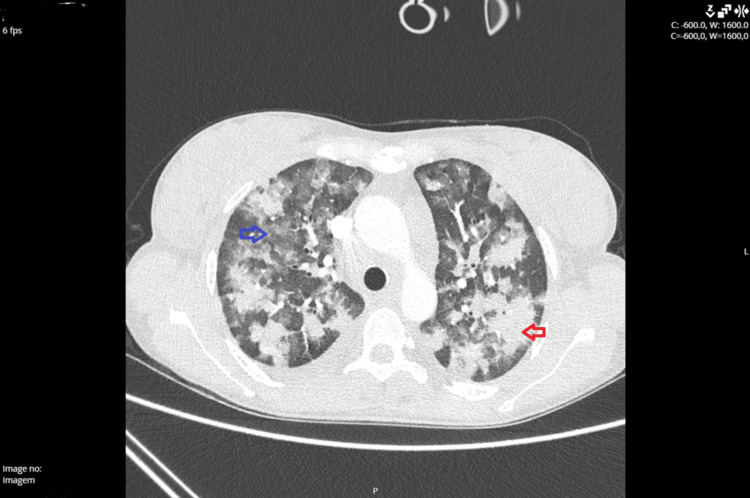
CT angiography showing extensive areas of consolidation (red arrow) and ground-glass densification (blue arrow) that raise the hypothesis of alveolar hemorrhage.
